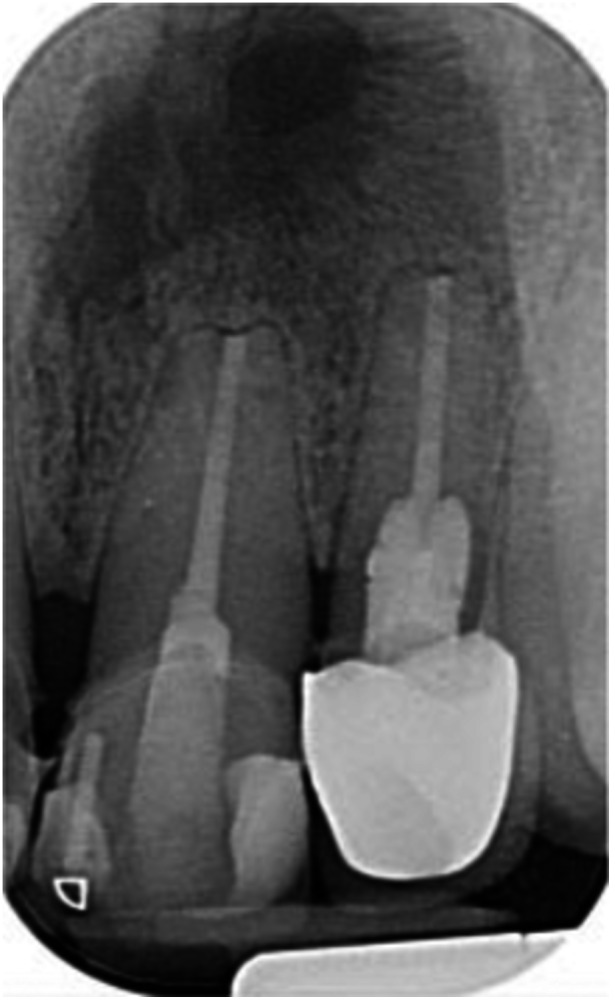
Case 2: The preoperative CBCT and periapical radiograph show an extensive periapical radiolucency involving teeth 21 and 22, extending very close to teeth 11 and 23, with a loss of the palatal cortical bone. Apical surgery was performed on teeth 21 and 22. At 6 months, marked healing can be seen, evidenced by a reduction in the periapical radiolucency size and reattachment of the PDL around the roots of teeth 21 and 22.
